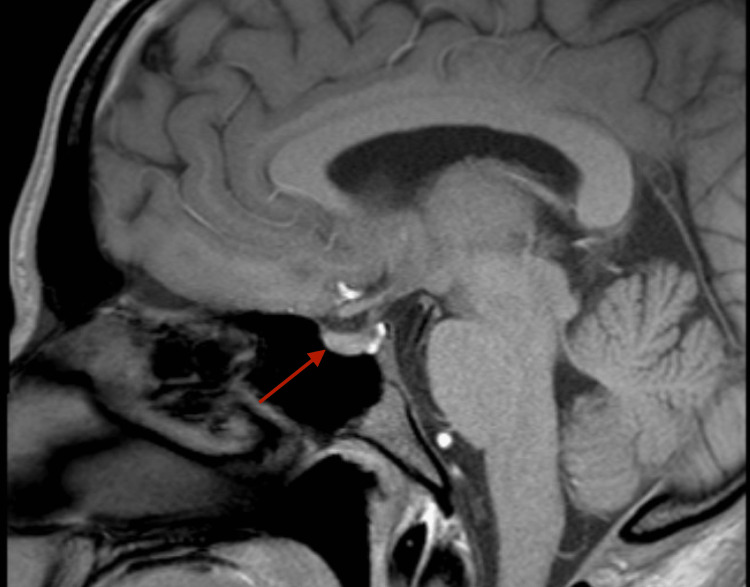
MRI Sella (pituitary with + without contrast)
